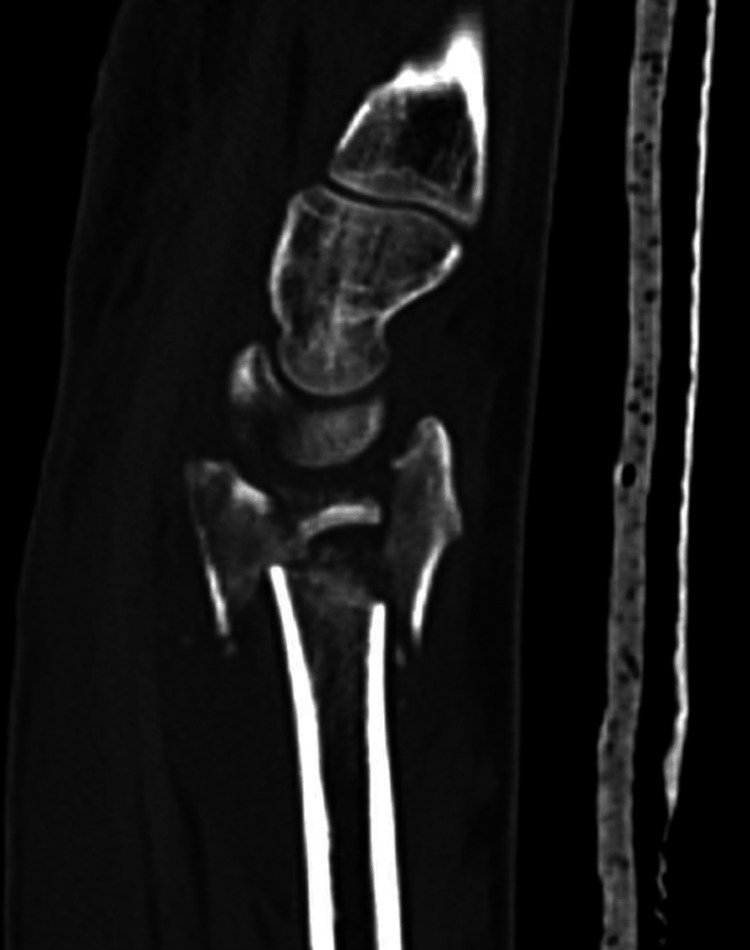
Sagittal CT reconstruction showing lunate fossa intercalary fragment rotated by 180 degrees.
